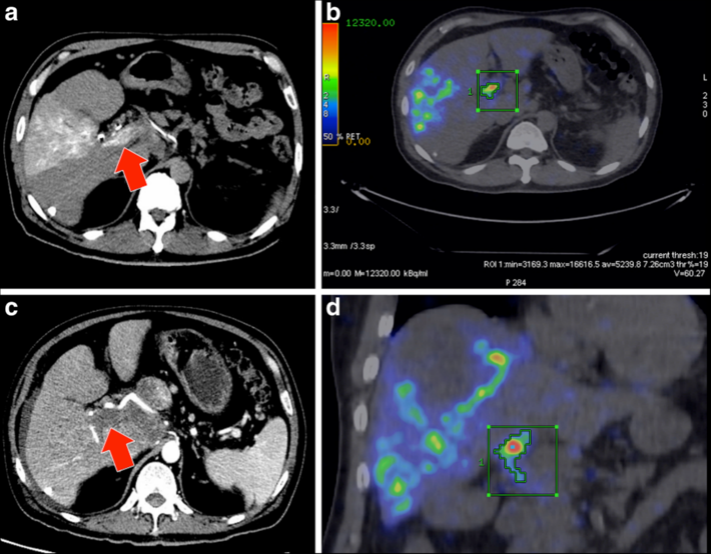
Patient 10. (a) Catheter-directed CT angiogram of the anterior branch of the right hepatic artery, supplying segments V and VIII, demonstrates contrast enhancement of a large portal vein tumor thrombus (arrow). (b, c) A ^90^Y PET VOI of the activity within the portal vein tumor thrombus was defined by volumetric isocontour thresholding, visually constrained to its anatomical margins as seen on the catheter-directed CT angiography. Within this VOI, the mean ^90^Y radioconcentration at the time of scan (5,239.8 kBq/ml) was decay-corrected back to the time of ^90^Y radioembolization (6,653 kBq/ml). By ^90^Y MIRD macrodosimetry, the mean absorbed dose of the portal vein tumor thrombus was approximately 316 Gy within the VOI. (d) Follow-up triphasic CT of the liver in the arterial phase at 4 months post-radioembolization demonstrates a complete lack of contrast enhancement within the VOI (arrow), suggesting a complete response and clinically validates the ^90^Y PET quantification.
